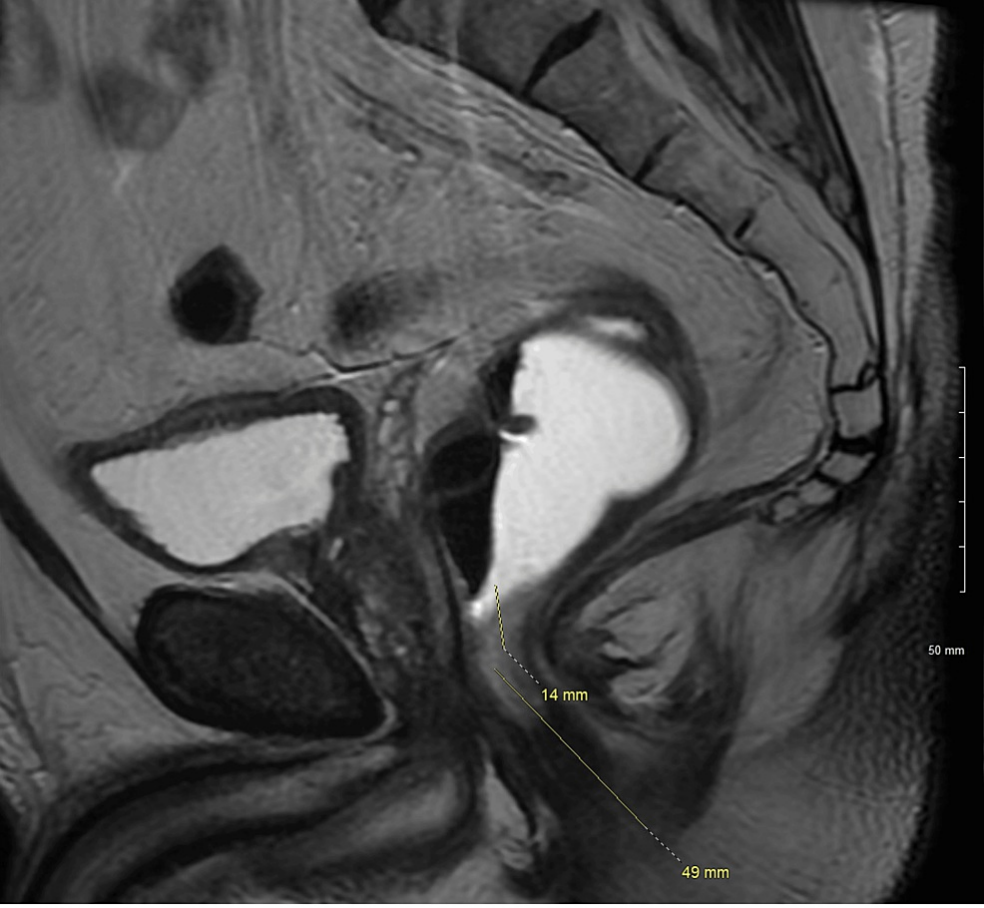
Sagittal MRI rectum with contrast showing the lowest extent of tumor distance from the anal verge: 6.5 cm. The lowest extent of the tumor is 1.4 cm from the top of the anal sphincter. Tumor: Less than 180 degrees of involvement of the rectal circumference, from 7 to 11 o'clock. The lesion is below the peritoneal reflection. No suspicious pelvic sidewall or extra mesorectal lymph nodes.
